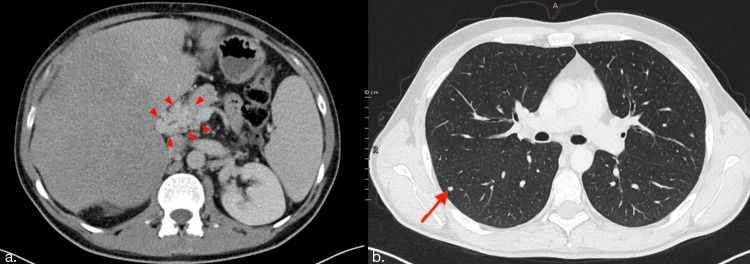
Axial abdominal CT scan venous phase (a) and thoracic CT scan in the pulmonary window (b) a: Peritoneal carcinomatosis is indicated by a red arrowhead. b: A suspicious pulmonary nodule is marked by a red arrow. CT: computed tomography
